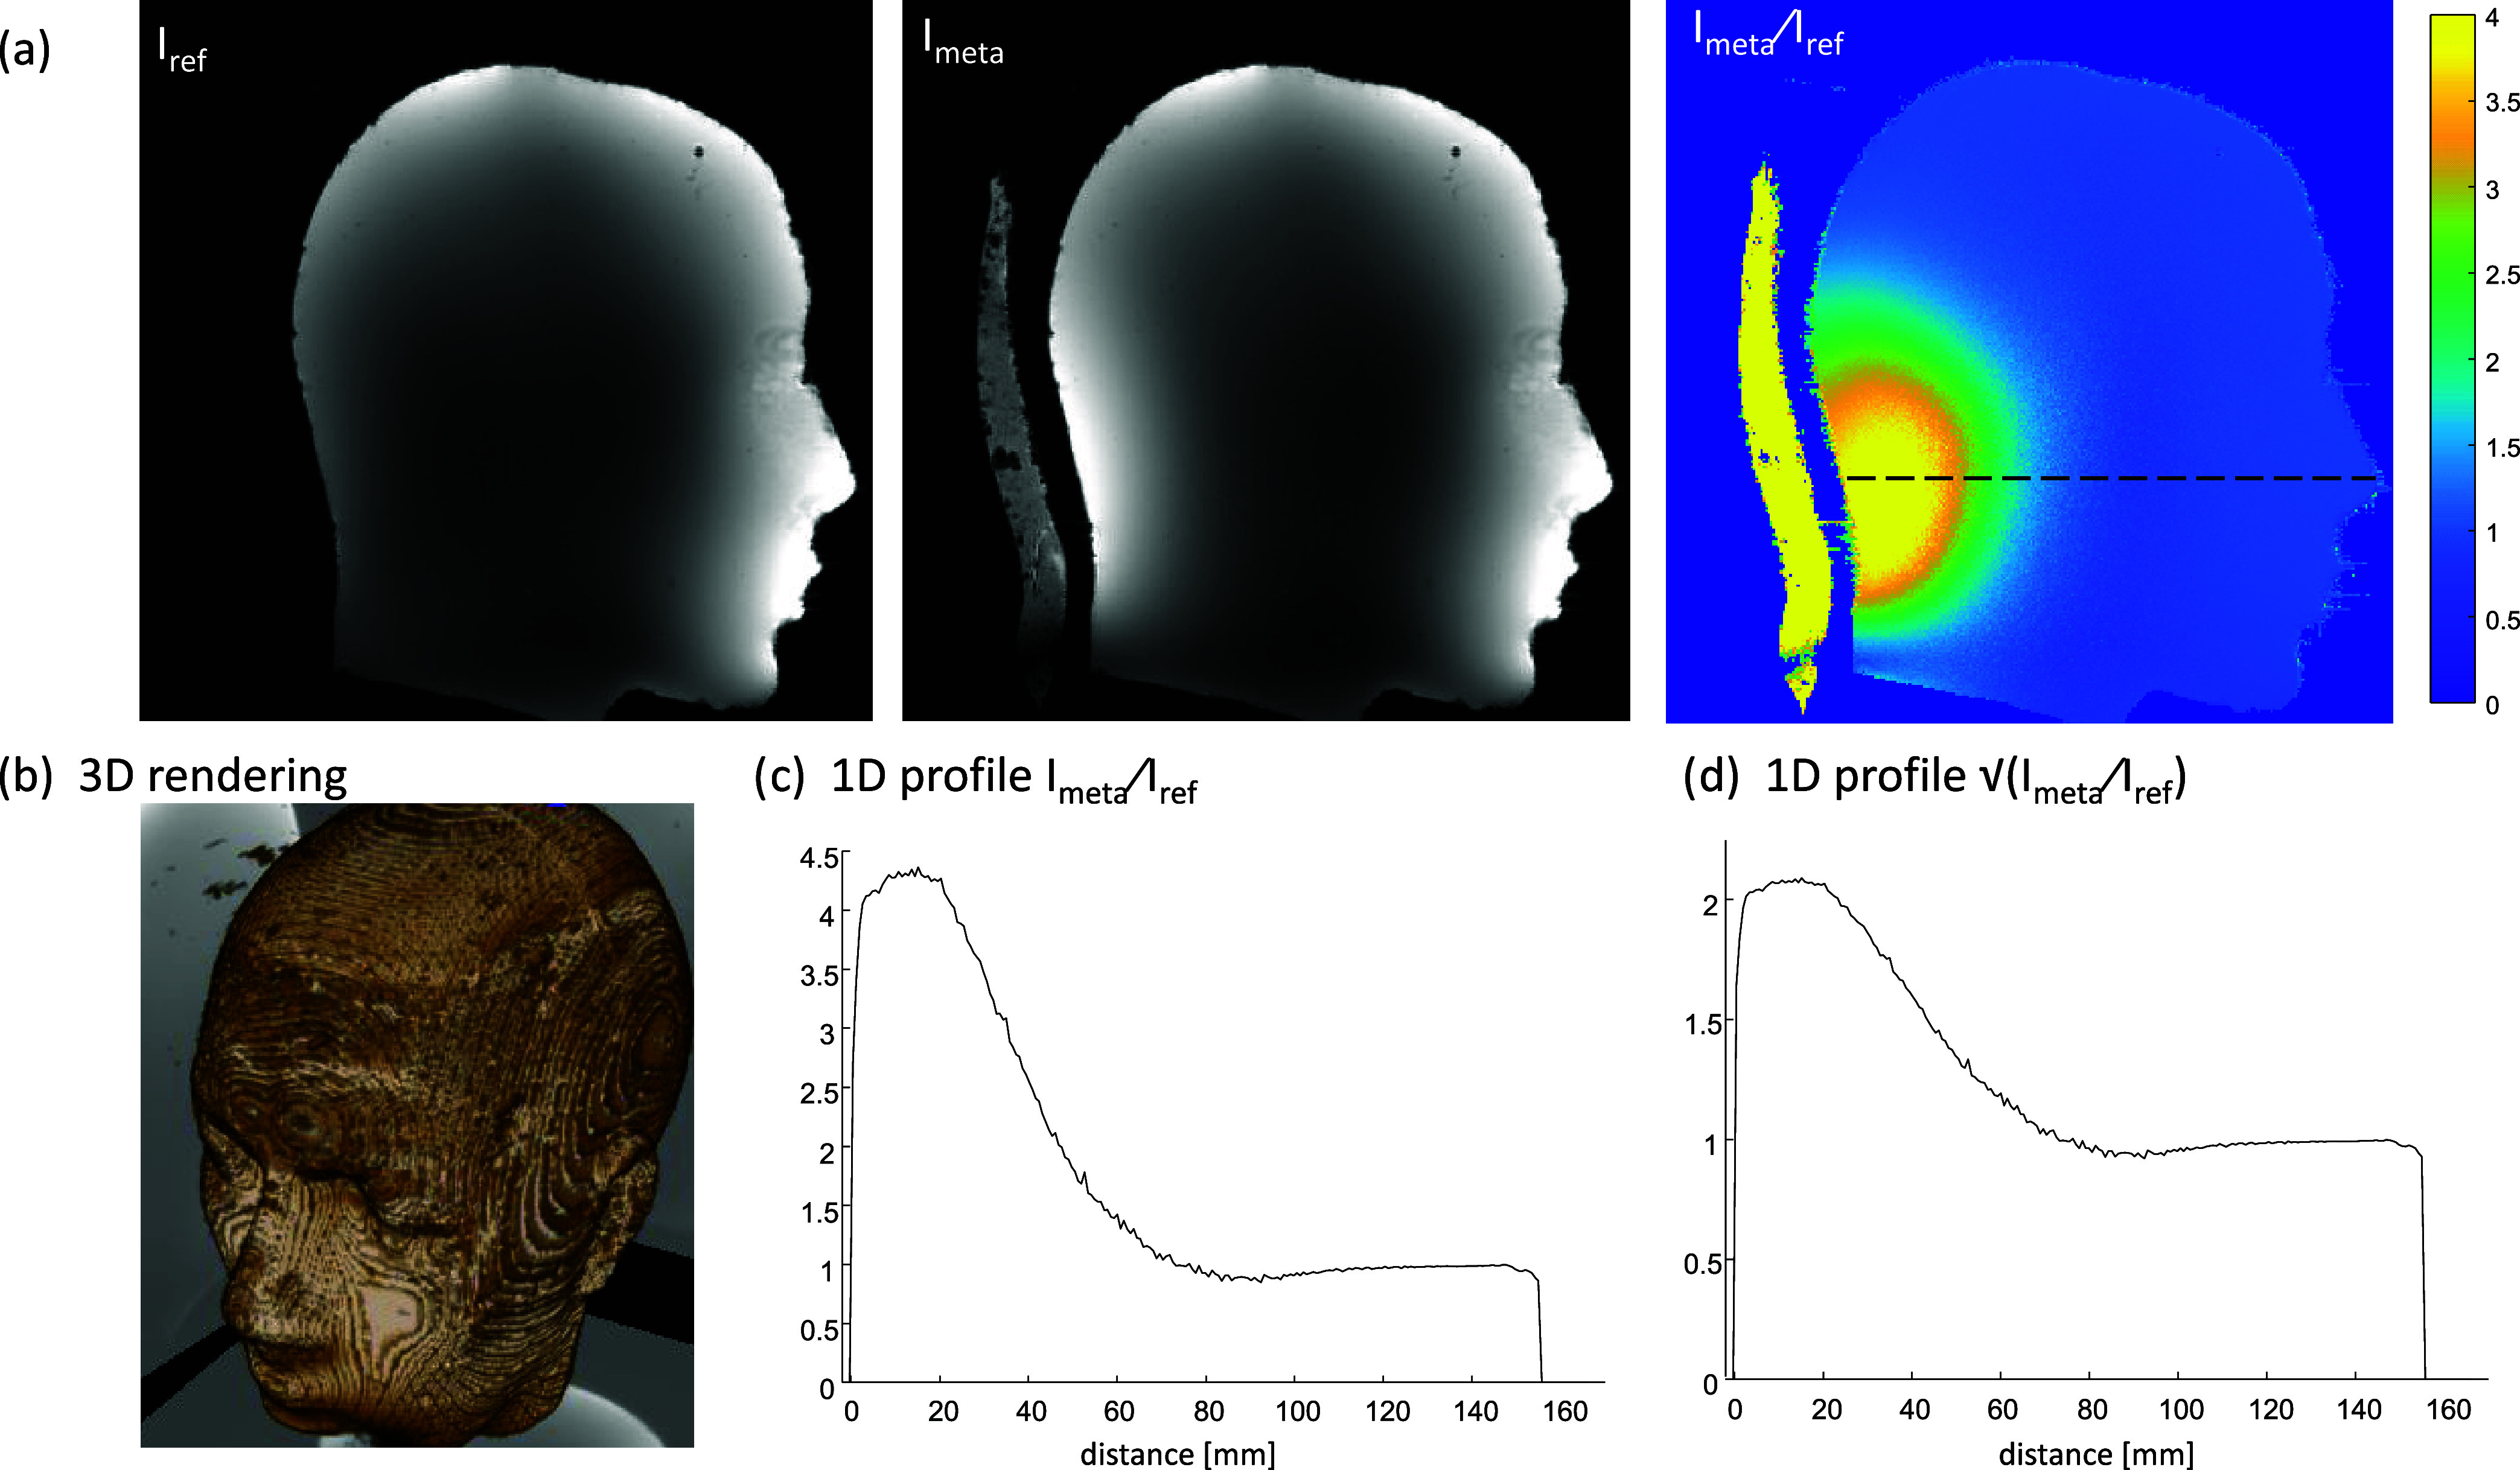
Sagittal MRI scan (gradient-echo) of a brain-mimicking
